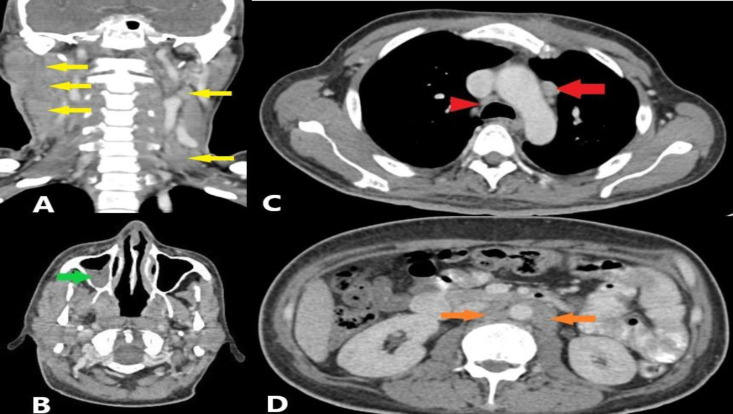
Contrast-enhanced CT scan of neck, thorax, and abdomen. A coronal image of the neck shows bilateral cervical nodes at levels II, III and IV (yellow arrow) (Figure A). Axial image at the level of nasopharynx shows no enhancing lesion seen at the nasopharynx or FOR region but the presence of air-fluid level in the right maxillary sinus secondary to chronic rhinosinusitis (green arrow) (Figure B). Axial image of the thorax depicts the right paratracheal (red arrowhead) and para-aortic LN (red arrow) (Figure C). Axial image at abdominal region shows para-aortic and aortocaval LN enlargement (orange arrow) (Figure D)
